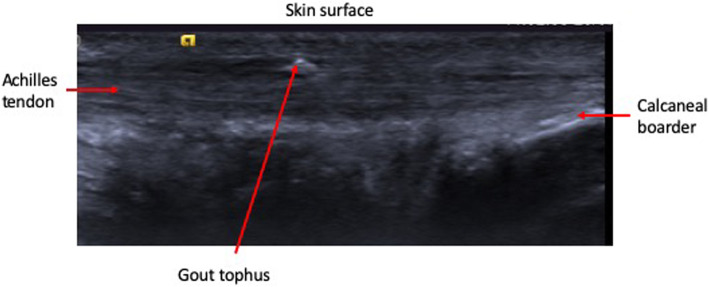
Gout tophus appearance in greyscale, conventional ultrasound
